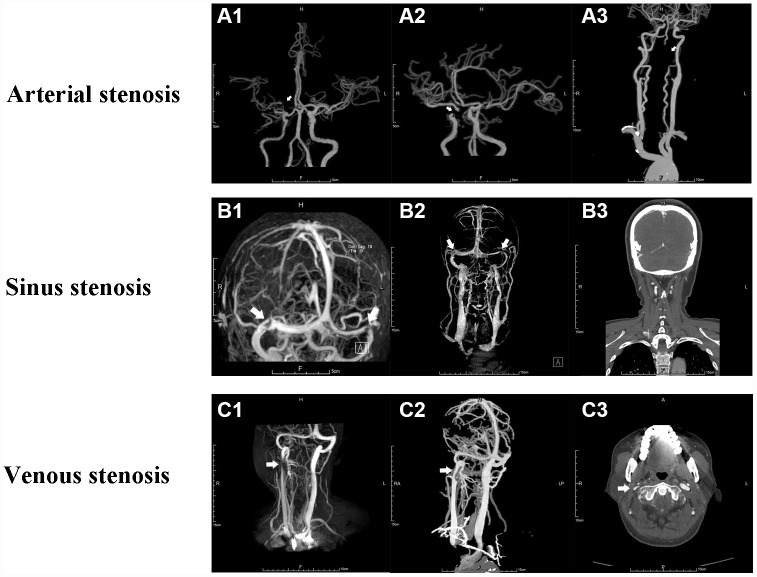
Angiography of cerebral arterial and venous stenosis. (A1–A3) Typical CAS represented by right MCA stenosis, right ICA stenosis and left VA stenosis on CTA respectively. (B1–B3) Bilateral cerebral transverse sinus stenosis presented on MRV and CTV. (C1-C3) Right IJVS J3 segment stenosis presented on MRV and CTV. The stenoses are indicated by arrows.
